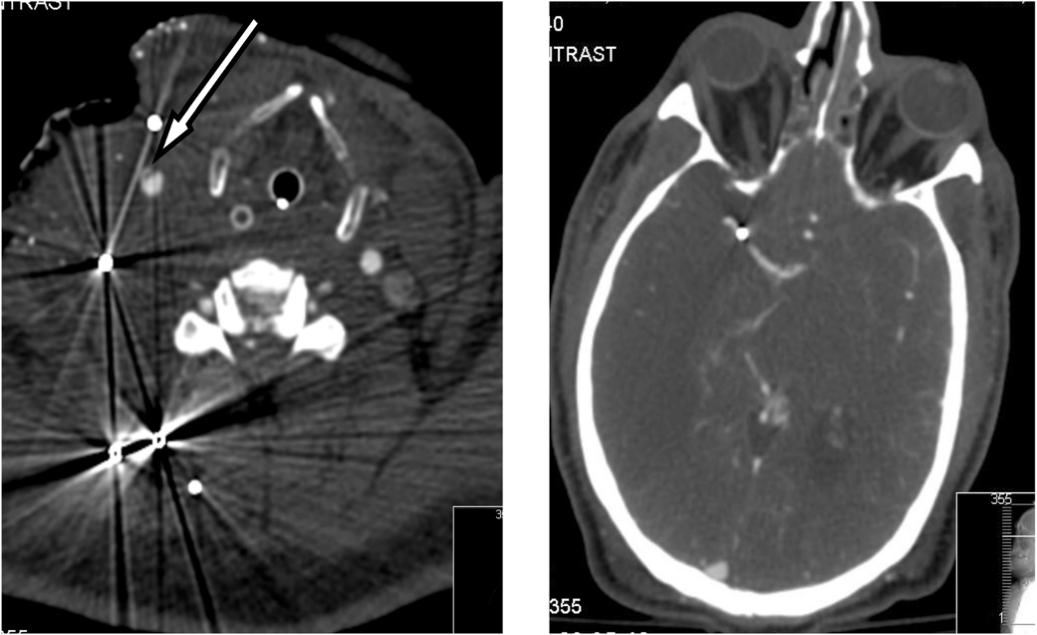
Axial images CT carotid and Circle of Willis angiogram.
